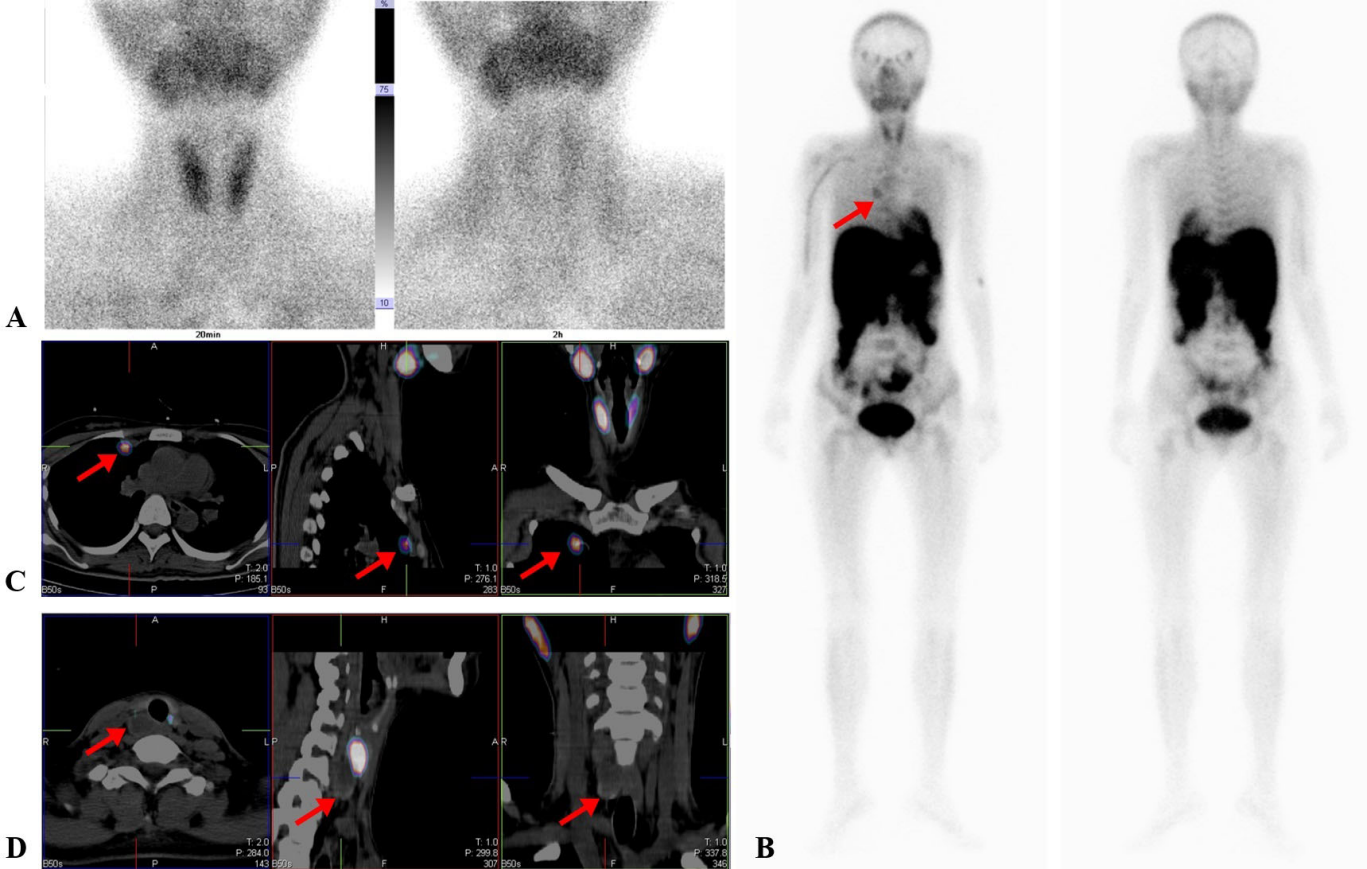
Dual-phase Tc-99m-sestamibi scintigraphy and SPECT/CT scans of the patient. (A) Early and delay imaging of dual-phase Tc-99m-sestamibi parathyroid scintigraphy. The thyroid gland was developed clearly in the early image and quickly decreased in the delayed image, whereas no radiotracer uptake was revealed at the lower pole of the right thyroid lobe both in early and delayed imaging. (B) Tc-99m-MIBI SPECT demonstrated a metastatic lesion in the right chest (arrow). (C) Tc-99m-MIBI SPECT/CT fusion images showed that high radiotracer uptake in the area of the right chest coincided with a mass in the upper right lung through CT scan (arrow). (D) Tc-99m-MIBI SPECT/CT fusion images showed that no survival of accumulation in the posterior of the right lower thyroid gland with low density (arrow).
